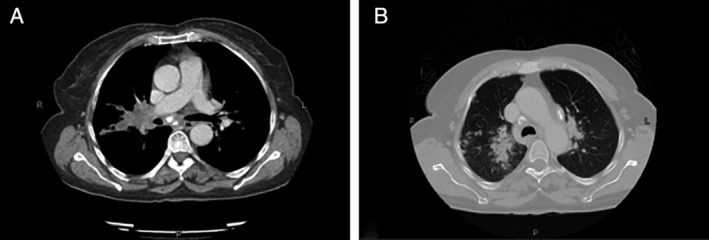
(A) Computed tomography (CT) scan of the chest mediastinal window displaying enlarged, calcified subcarinal lymph node. (B) Lung window showing right‐sided lung parenchymal infiltrates.
